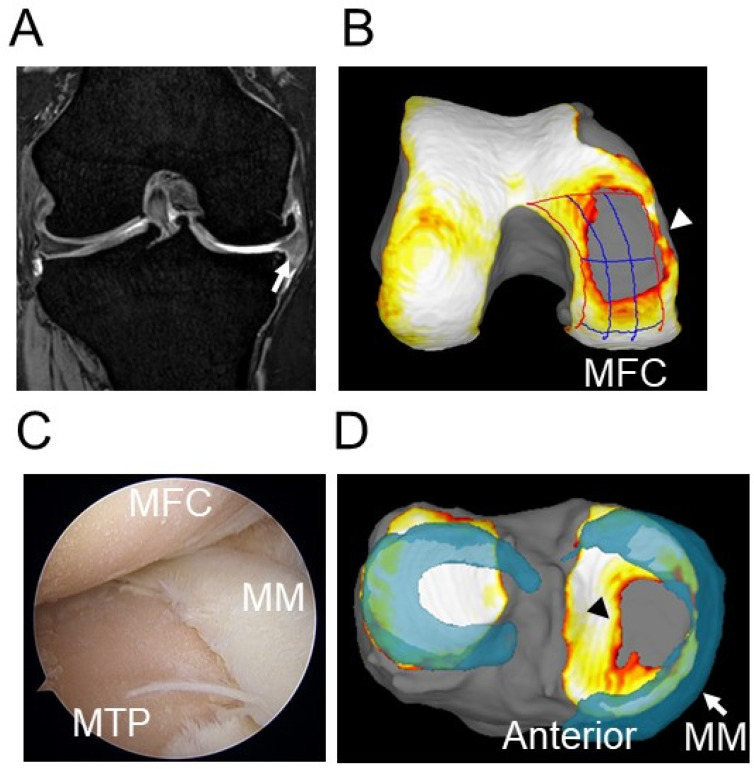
An extruded medial meniscus in the right knee. (A) Medial meniscal extrusion was confirmed in a coronal view (white arrow). Cartilage defects were observed in the medial compartment. (B) The 3D MRI view shows the cartilage defect in the MFC (white arrowhead). (C) Arthroscopic findings indicate decreased meniscal coverage of the MTP and cartilage defects in the MFC and the MTP. (D) The 3D MRI view shows extrusion of the MM (white arrow) and a cartilage defect in the MTP (black arrowhead). MM, medial meniscus; LM, lateral meniscus; MFC, medial femoral condyle; MTP, medial tibial plateau.
